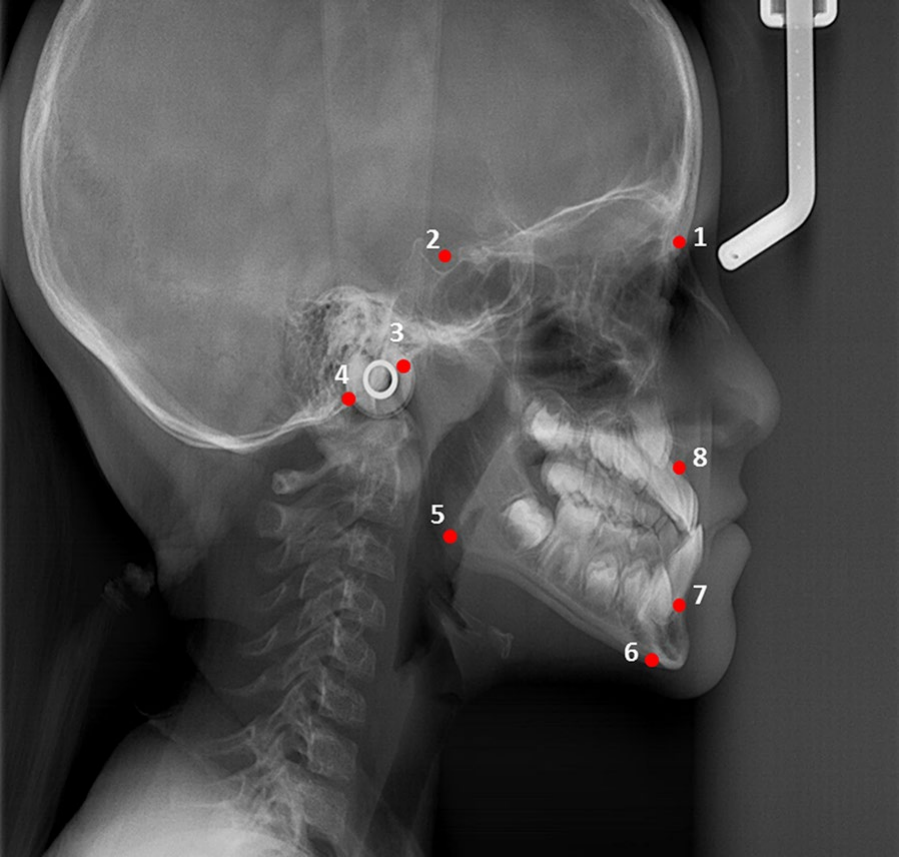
Location of reference points for lateral cephalic radiograph obtained for this study. *1* Nasion (N): junction of nasal and frontal bones. *2* Sella (S): midpoint of sella turcica. *3* Articular (Ar): point of intersection of the basilar apophysis of the occipital bone and the posterior border of the condyle. *4* Basion (Ba): most anterior inferior point of the anterior border of the occipital hole. *5* Gonion (Go): determined at the bisection of the angle formed by the posterior surface of the mandibular ramus and the mandibular body. *6* Menton (Me): lower most point of the mandibular symphysis curve. *7* B point (B): deepest point on anterior profile of mandibular symphysis. *8* A point (A): deepest point on anterior profile of superior maxilla
